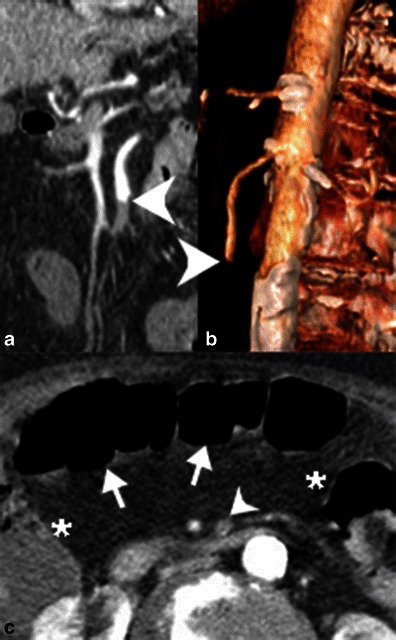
Embolic occlusion of the superior mesenteric artery. a Contrast-enhanced coronal reformatted CT image and b 3D volume-rendered reconstruction of demonstrate an abrupt cutoff of the proximal superior mesenteric artery (*white arrowheads*) secondary to an embolus. c Axial contrast-enhanced CT demonstrates relatively poor enhancement of the transverse colon (*white arrows*) relative to the hepatic and splenic flexures (*). Note also the occluded SMA (*white arrowhead*)
